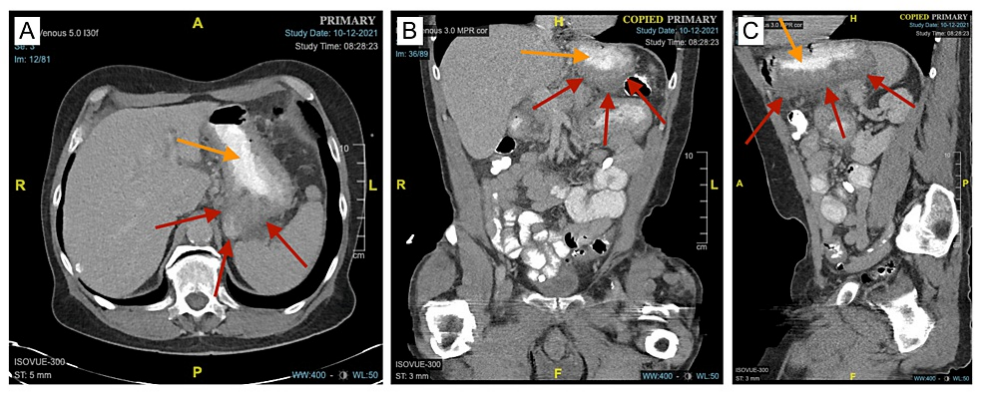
CT of the abdomen and pelvis with oral contrast one month after the initial discharge A, B, C: Axial, coronal, and sagittal views showing interval improvement and a decrease in the overall size of the GIH (red arrows). Due to the reduced size of the GIH, the lumen of the stomach (orange arrows) is less compressed. GIH: gastric intramural hematoma
